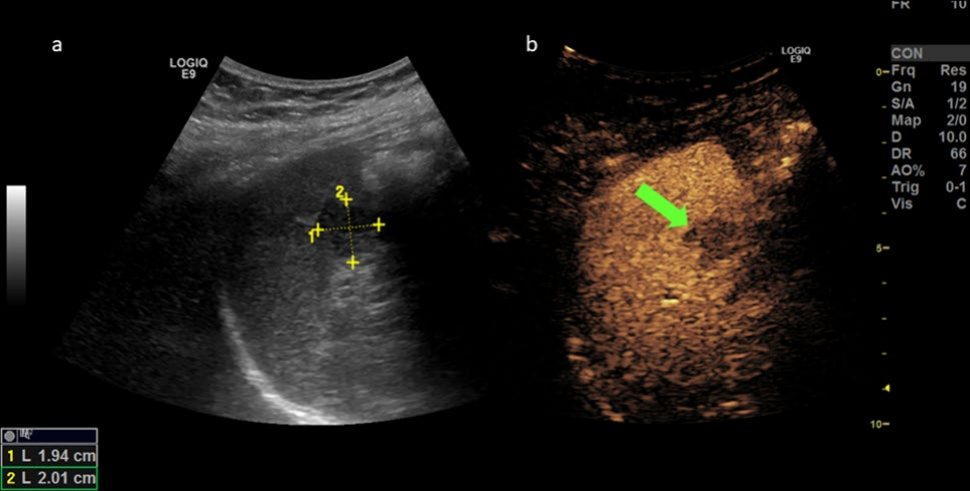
Ultrasound (US) scan of the splenic hypoechoic lesion located near the hilum (2a). Fast wash-out in the venous phase at contrast enhanced ultrasound (CEUS) (2b, the arrows show wash-out in venous phase on B-mode and CEUS)
